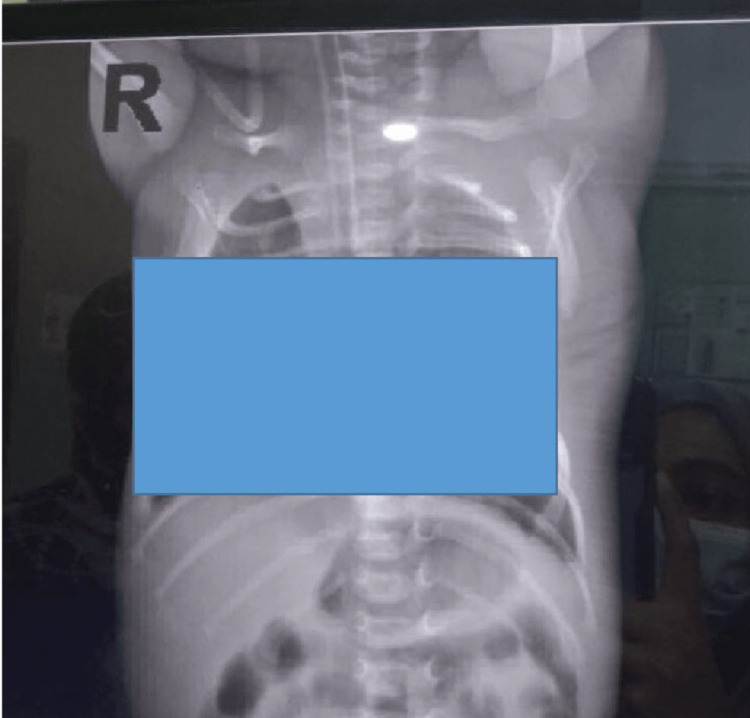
CXR image masking the lung fields. CXR: chest x-ray
